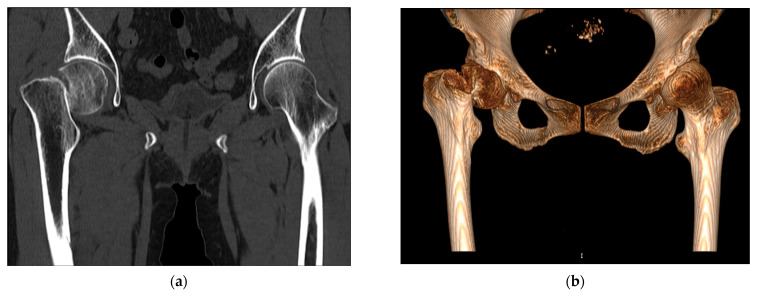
Intracapsular fracture occurring because of low-energy falls in the elderly: (a) CT diagnostic image; (b) 3D reconstruction model.
